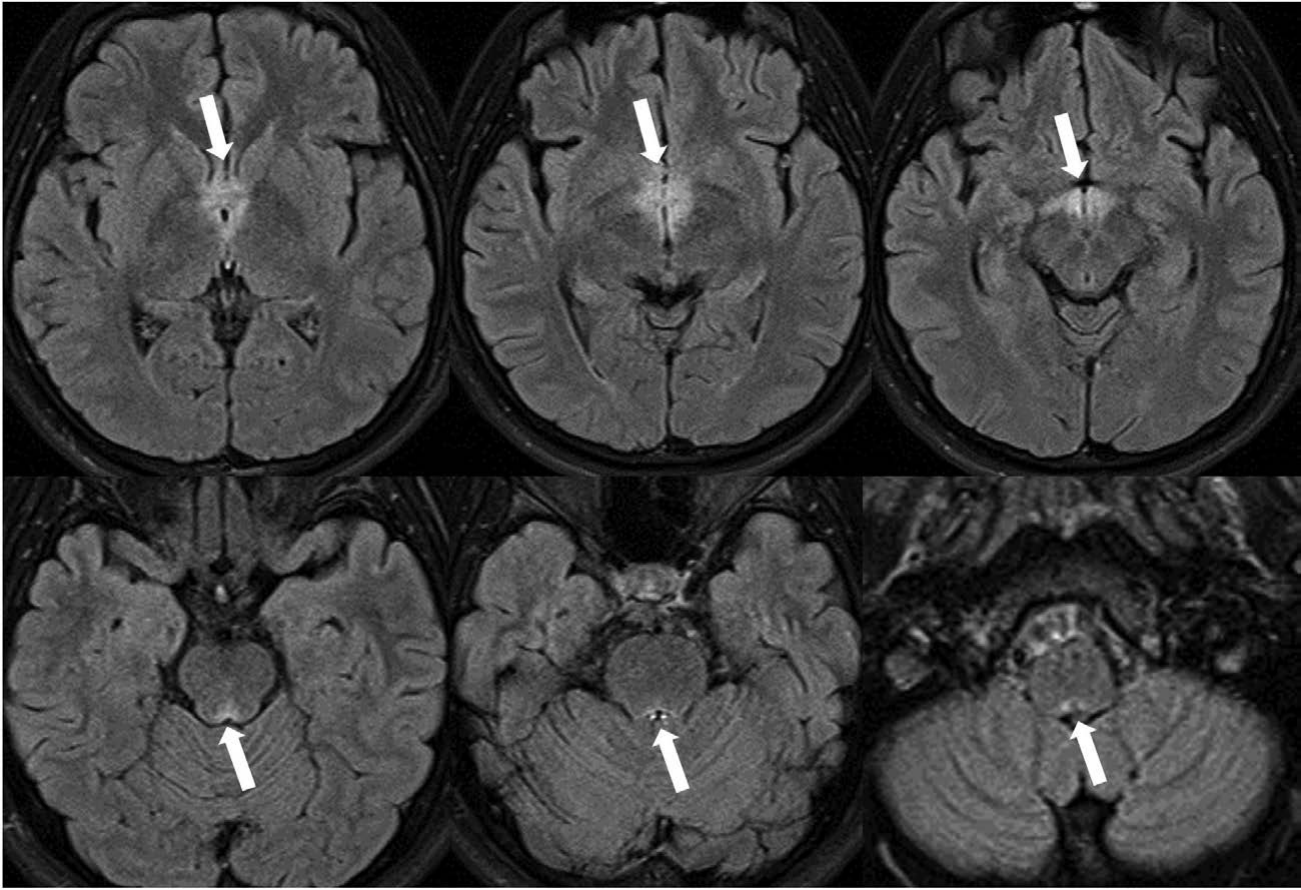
Brain magnetic resonance imaging (MRI) follow-up in the 2^nd^ wk of hospitalization. Fluid-attenuated inversion recovery (FLAIR) images show the hyperintense lesions in the hypothalamus, periaqueductal area, periependymal surface of fourth ventricle and area postrema. The hyperintensity lesions are more pronounced compared with the initial brain MRI.
